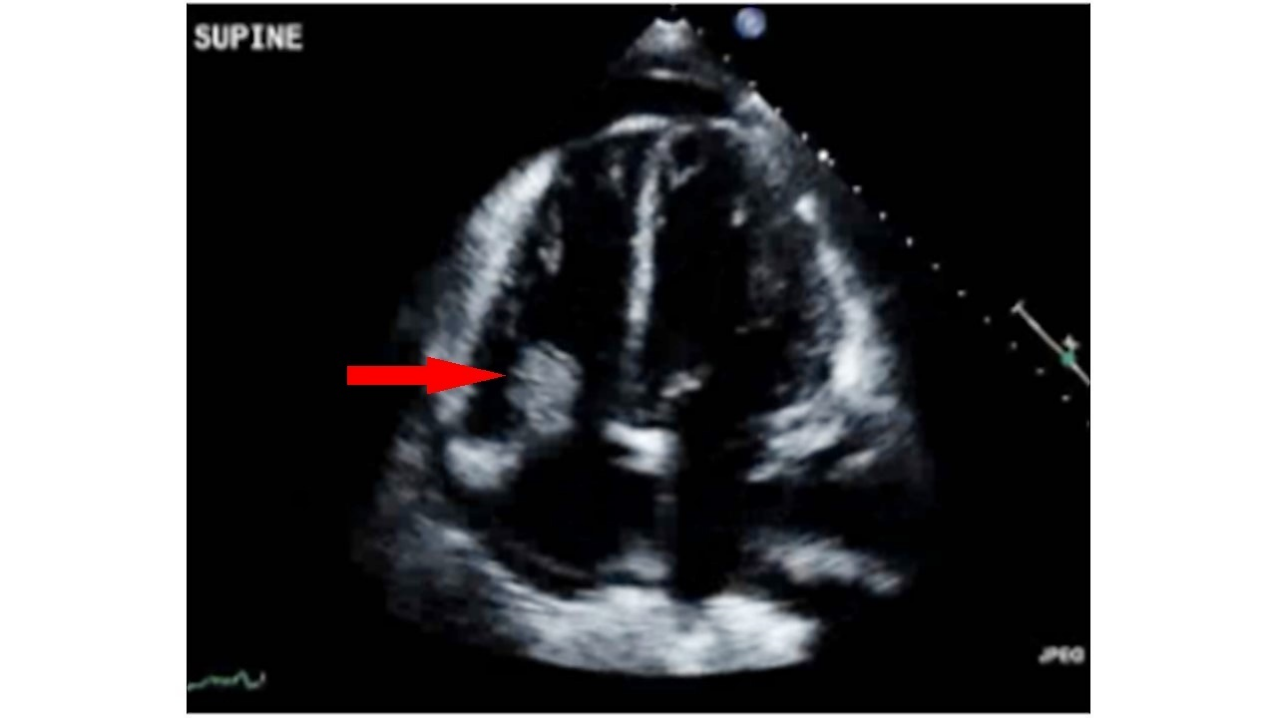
Transthoracic Echocardiogram A large mobile echodensity (2.65 x 1.52 cm) attached to the tricuspid valve leaflet that attaches to the RV free wall. It is homogeneous, globular, and moves freely between the RA and RV.
